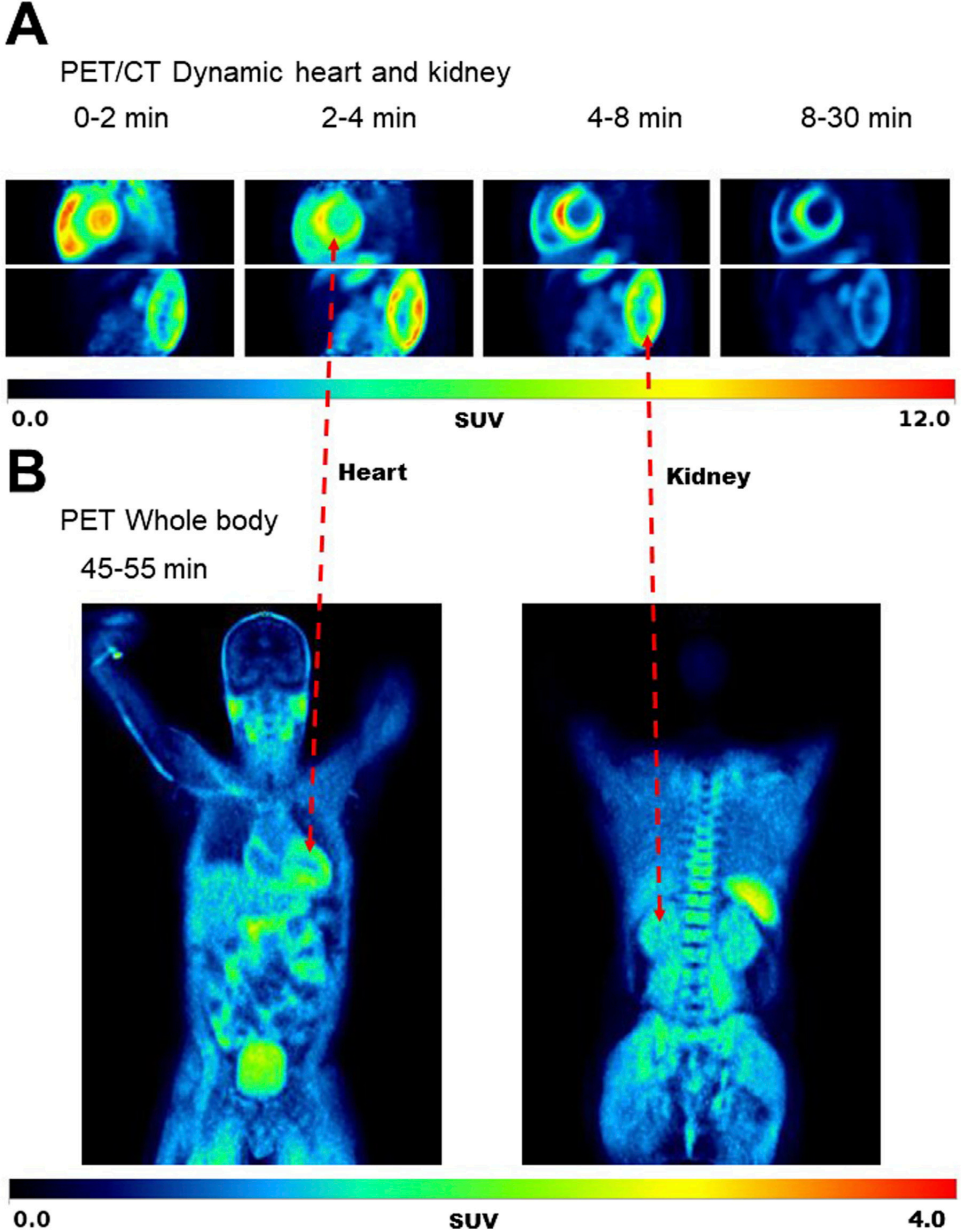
Typical images for the dynamic scan of the heart and kidney (A) and whole body (B) during the Fasted condition. SUV – standardized uptake value.
